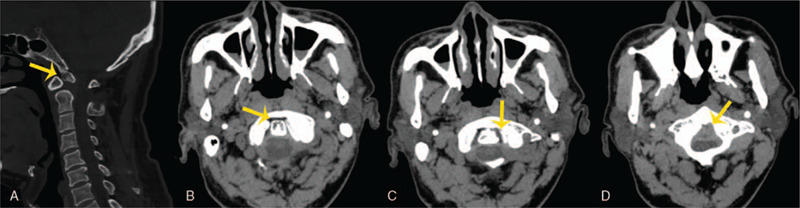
Three-dimensional CT. (A) The base and apex of the odontoid process were separated; (B–D) the left and right atlantoaxial space are narrow and partially fused.
